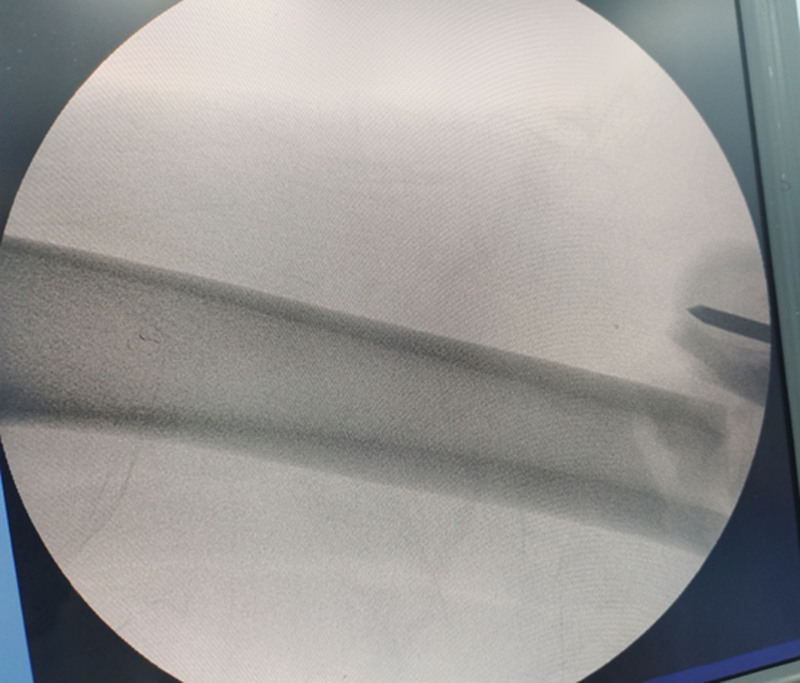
Intraoperative lateral radiograph showed the length recovery after traction, but there was anterior-posterior lateral displacement.
